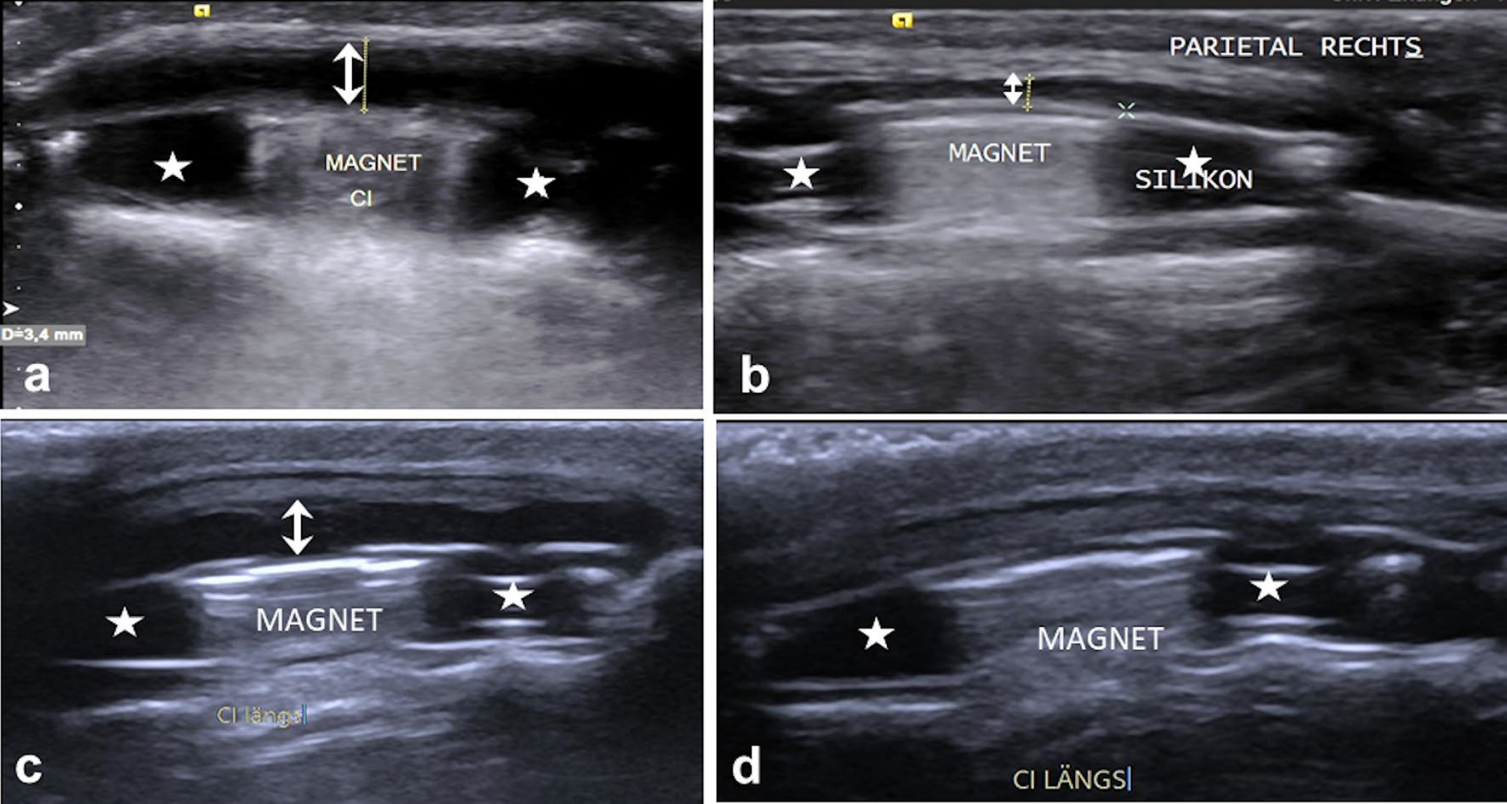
Patients with haematoma and seroma around their CI marked by double arrows. The anechoic magnet housing is indicated by asterisks; the implant magnet is indicated by “MAGNET”. a 4-year-old child implanted with a CI24RE (CA) on the right side diagnosed with haematoma around the CI after trauma; an anechoic area over the implant is measured at 3.4 mm in height; the haematoma was aspirated and a pressure bandage and oral antibiotics were administered. b Follow-up ultrasound showed successful reduction of the haematoma over the implant to 1.3 mm 9 days later. c A 16-year-old patient with a CI24RE (CA) on the right side diagnosed with a seroma around the device that was measured at 2.5 mm in height. The patient was advised not to use his device temporarily. d Follow-up ultrasound 17 days later showed no seroma
